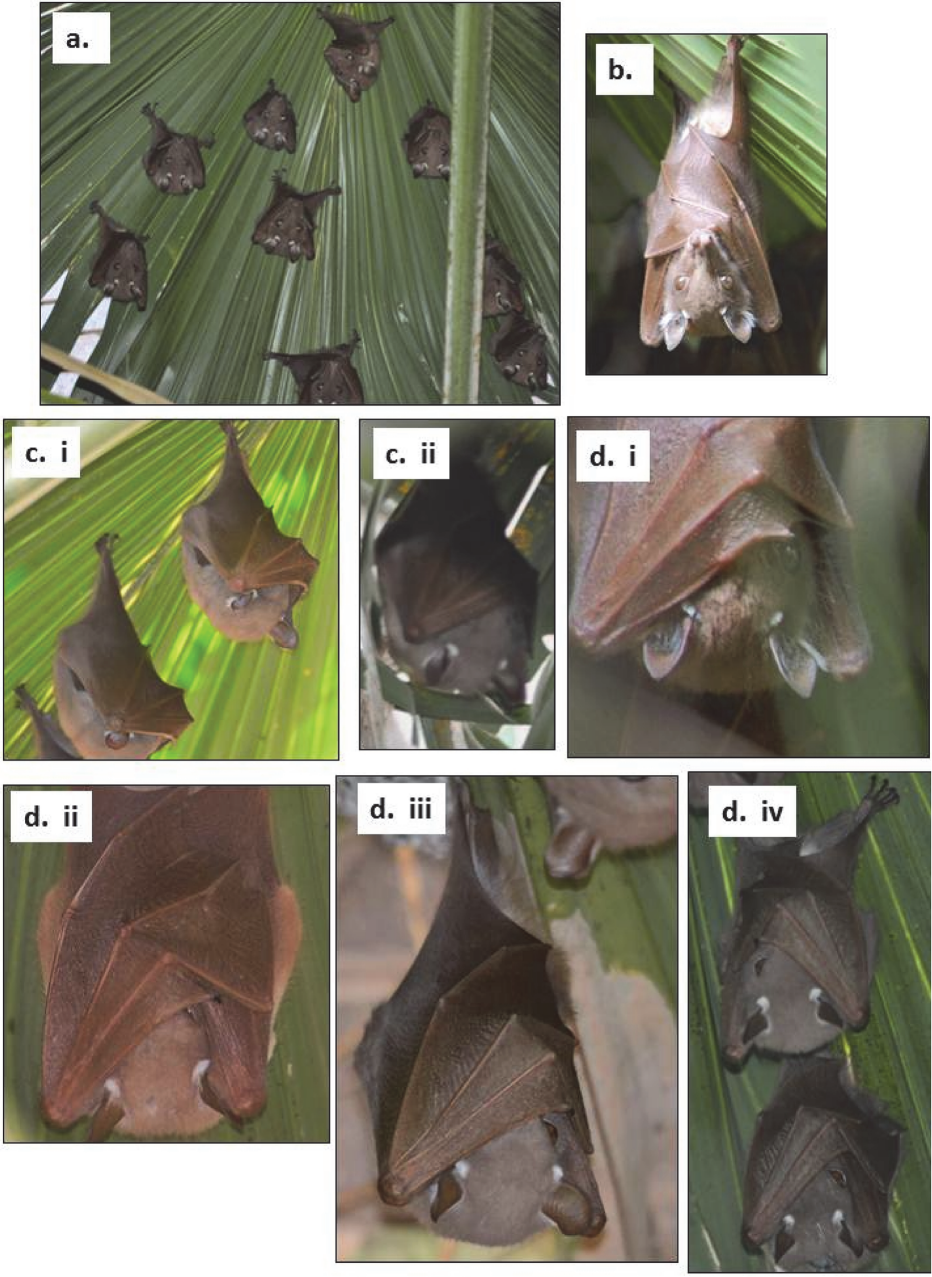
Photographic examples of *E*. *wahlbergi* roosting and showing different non- and sleep behaviours (see methods) where a. bat roost; b. awake bat with both eyes open; c. bats sleeping with both eyes closed, (bats sleep usually with wings wrapped around and covering both eyes), and d. bats sleeping with one eye closed. (Captured using a Nikon D5000 digital camera, copyright CT Downs).
